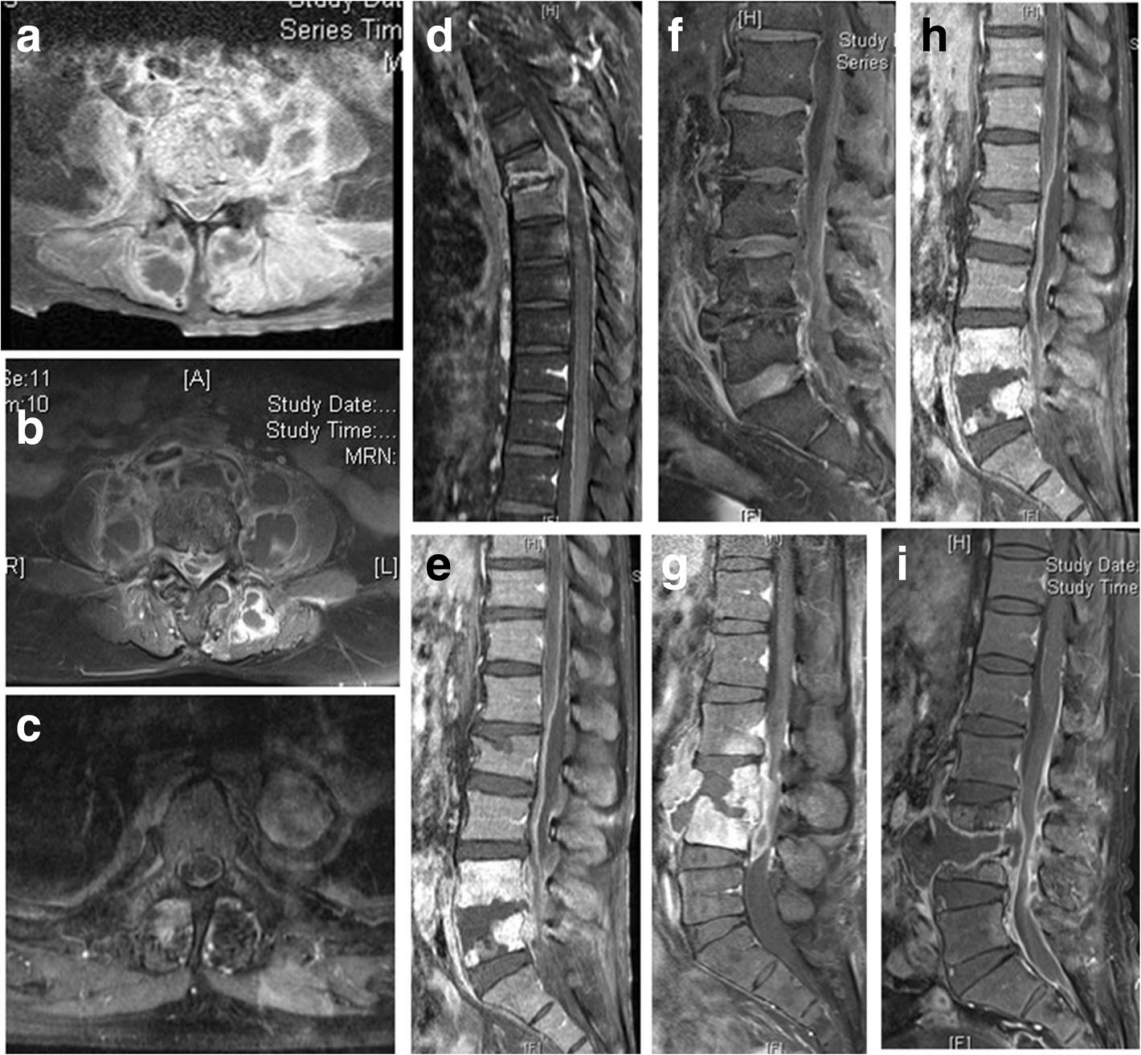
Elucidation of epidural abscess and muscle abscess in contrast-enhanced magnetic resonance imaging with fat suppression among 9 g-positive pyogenic spondylodiscitis (a–c). The spinal epidural abscess was defined as an epidural mass with iso-or hypointensity on T1-weighted images, which was surrounded by linear enhancement (ring sign) on magnetic resonance imaging. Abscess formation in psoas muscle or back muscle was defined as an asymmetrical enlarged mass of the involved muscle with ring sign on magnetic resonance imaging. d–f Ventral epidural abscess was noticed on sagittal gadolinium-enhanced fat-suppressed T1-weighted magnetic resonance
